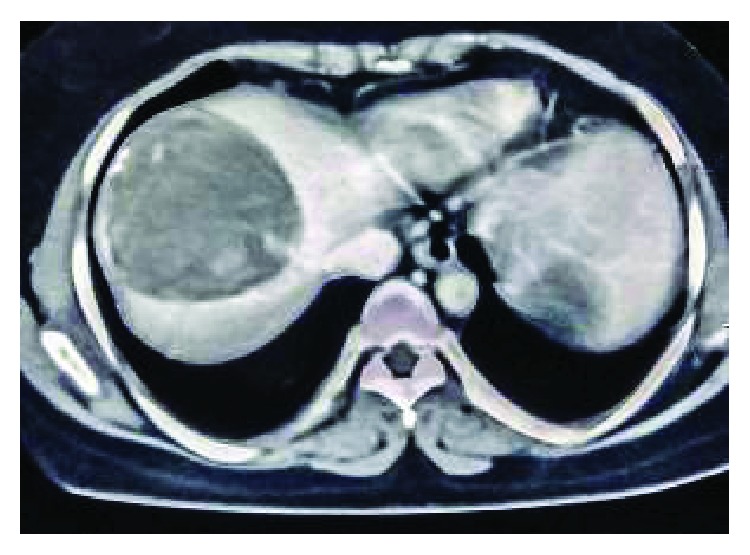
CT scan of abdomen showing giant lobulated hydatid cyst in the right lobe of the liver.
